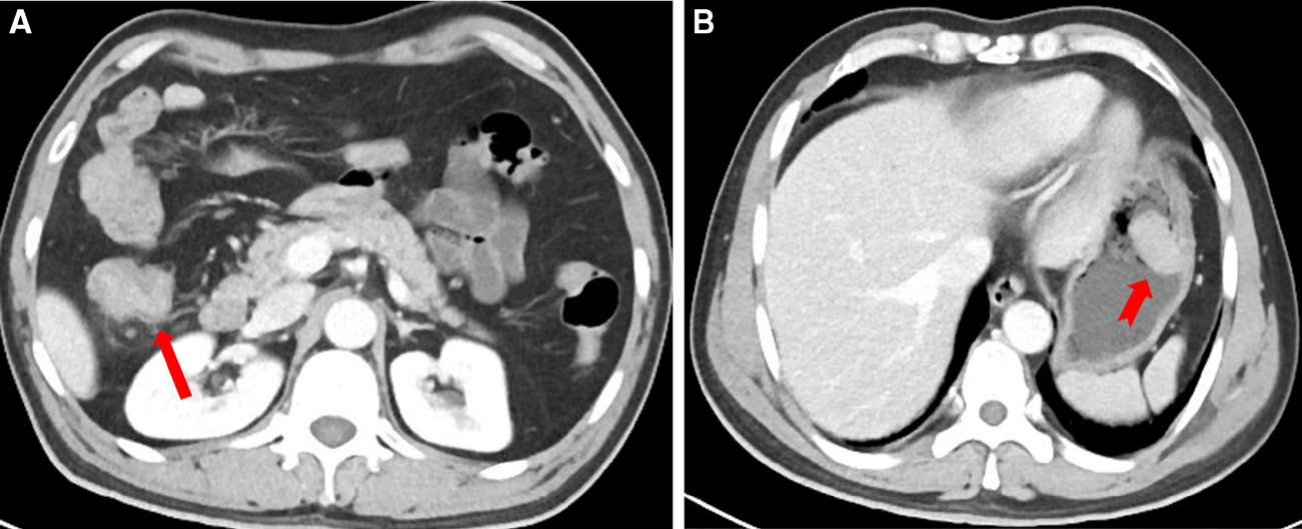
Computed tomography images from the case of a 45-year-old man with synchronous isolated gastric metastasis. (A) Contrast-enhanced CT image shows the thickened wall (red arrow) in the ascending colon persistent significant enhancement in the portal venous phase. (B) Contrast-enhanced CT image shows a polypoid mass (red dovetail arrow) with persistent enhancement in the portal venous phase.
